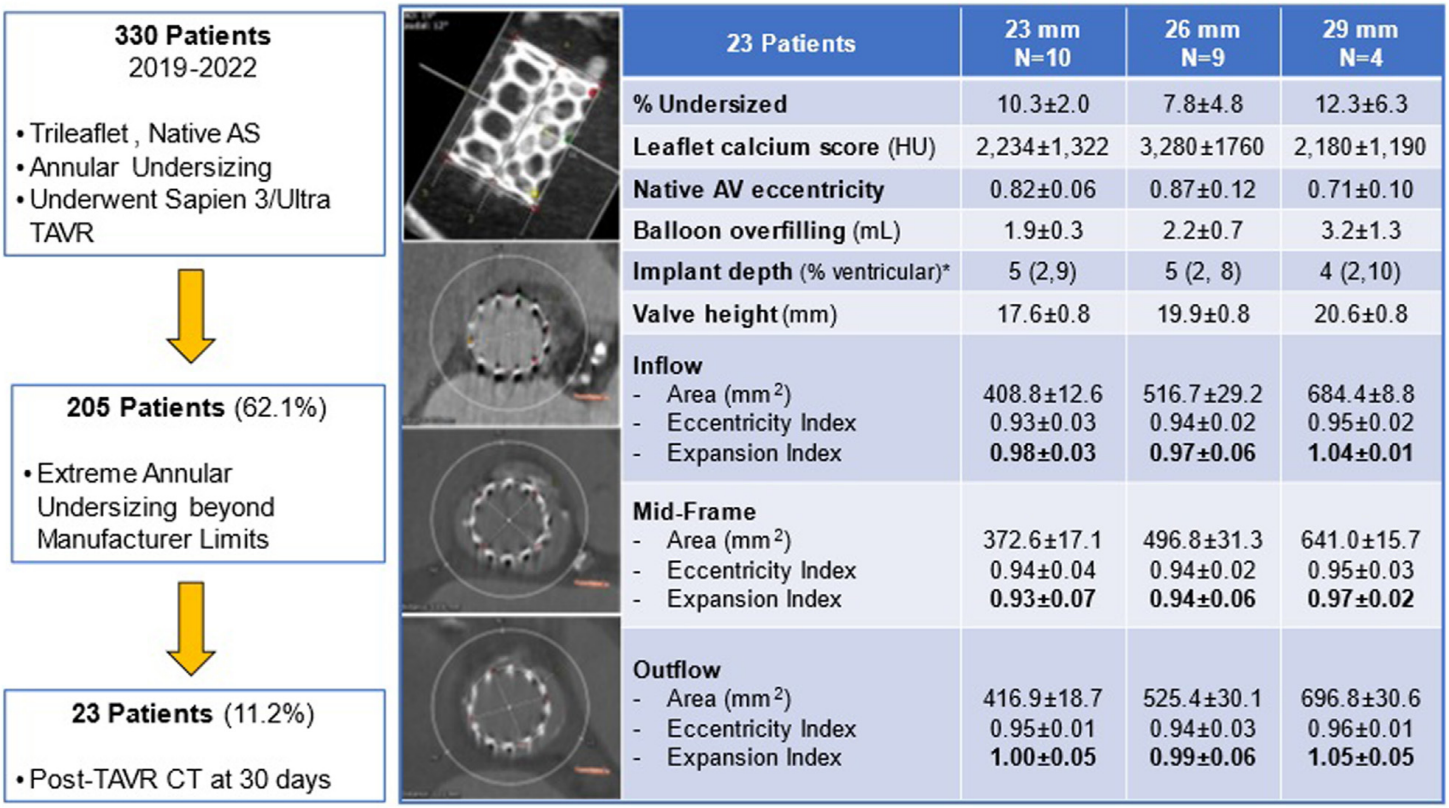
Summary of our post-TAVR computed tomography (CT) study on balloon overfilling in SAPIEN 3 TAVR in extremely undersized anatomies. AS, aortic stenosis; AV, aortic valve; HU, Hounsfield units; TAVR, transcatheter aortic valve replacement. ∗Represented by median and interquartile range.
